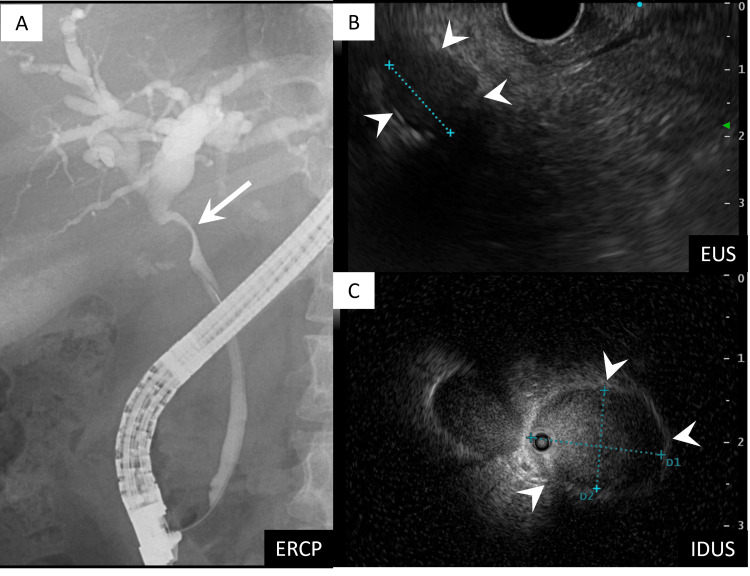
Findings of endoscopic retrograde cholangiopancreatography (ERCP), endoscopic ultrasound(EUS), and intra-ductal ultrasound (IDUS). (A) ERCP shows a stenotic lesion at the confluence of the three bile ducts, caused by extrinsic compression resembling a submucosal tumor (arrow).
